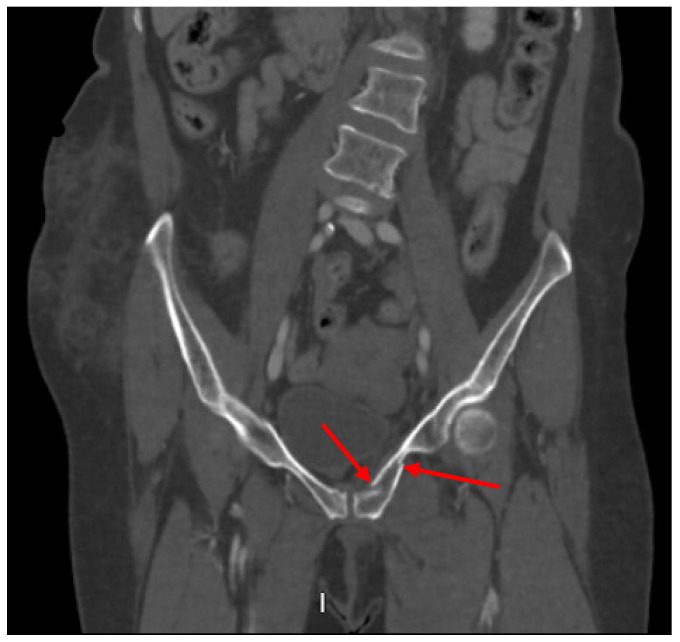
CT abdomen/pelvis images of the described fractures in case 2. Red arrows are used to indicate the location of pelvic fractures for easier identification.
